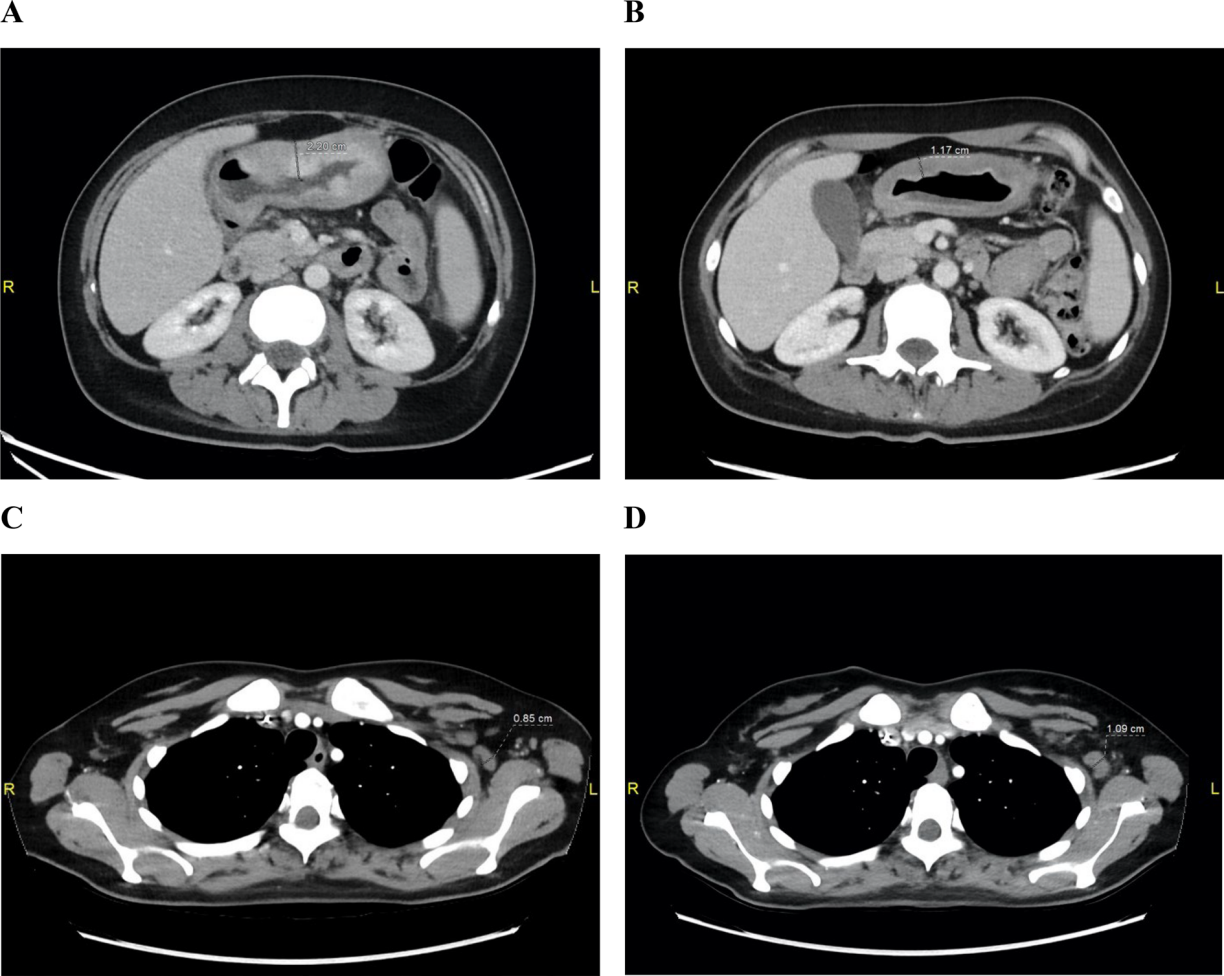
Contrast-enhanced CT imaging at baseline and during systemic treatment (A) Baseline CT demonstrating diffuse thickening of the gastric wall in the antrum and pylorus, measuring up to approximately 2.20 cm; (B) Follow-up CT performed in January 2025 during systemic treatment with zolbetuximab in combination with oxaliplatin and 5-FU, showing partial remission with regression of gastric wall thickening in the antrum and pylorus to 1.17 cm; (C) Follow-up CT performed in January 2025 demonstrating a left axillary lymph node with a short-axis diameter of 0.85 cm; (D) Follow-up CT performed in May 2025 during ongoing systemic treatment, showing progressive enlargement of left axillary lymph node metastases with an increase in short-axis diameter to 1.09 cm
